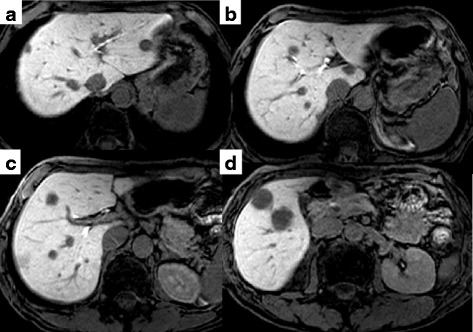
Gd-EOB-DTPA-enhanced MRI on hepato-biliary-phase findings of CRLM after the induction chemotherapy. a–d The volume of bilateral CRLM slightly downsized and the tumor border turned to be clear on hepato-biliary-phase images of EOB-MRI
